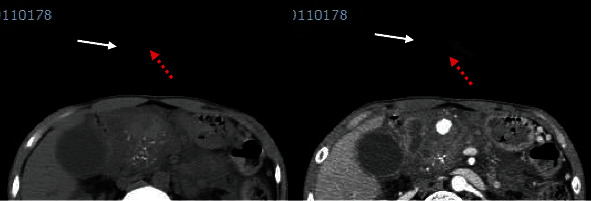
Axial contrast-enhanced computed tomographic images demonstrate a second pseudoaneurysm arising from the gastroduodenal artery, suggesting recent and ongoing bleeding (white arrow: pancreatic pseudocyst; black arrow: pseudoaneurysm filling with contrast; red dashed arrow: thrombus).
